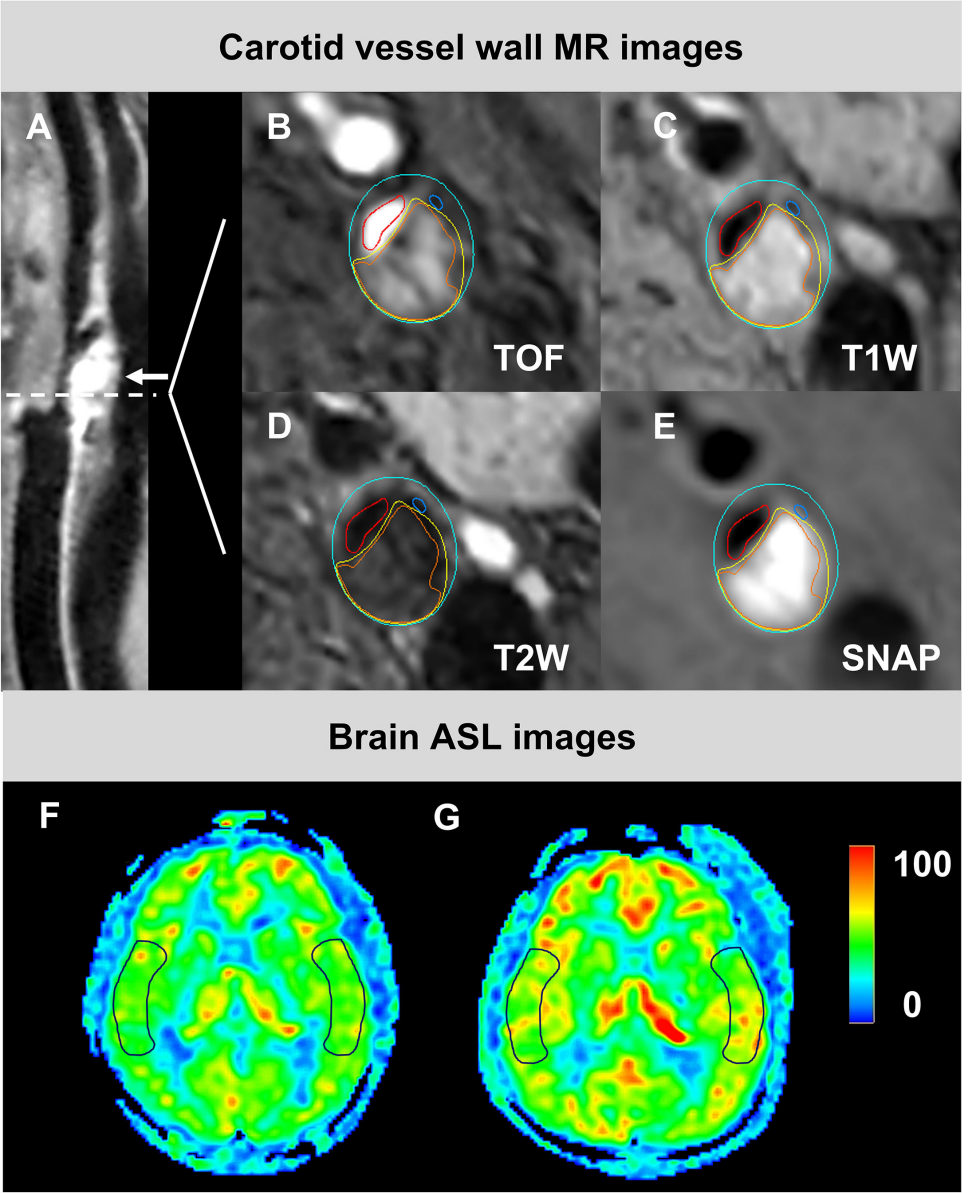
The images from a 85-year-old male patient who had carotid atherosclerotic plaque with IPH and calcification in the left side and changed CBF undergoing CEA. An eccentric plaque (white arrow) was delineated in the curved projection reconstructed image of 3D T1W (A). IPH (orange), calcification (navy blue), and LRNC (yellow) were demonstrated on the axial multi-contrast MR images with the manually outlied lumen (red), wall (blue), and component boundaries (B–E). The preoperative (F) and postoperative (G) CBF maps were shown, respectively. The ROIs on images (F,G) were located at the cortical flow territory of the middle cerebral artery on both index and contralateral sides. CBF, cerebral blood flow; CEA, carotid endarterectomy; IPH, intraplaque hemorrhage; LRNC, lipid-rich necrotic core; ROIs, region of interests; SNAP, Simultaneous non-contrast angiography intraplaque hemorrhage; T1W, T1-weighted; T2W, T2-weighted. TOF, time-of-flight.
